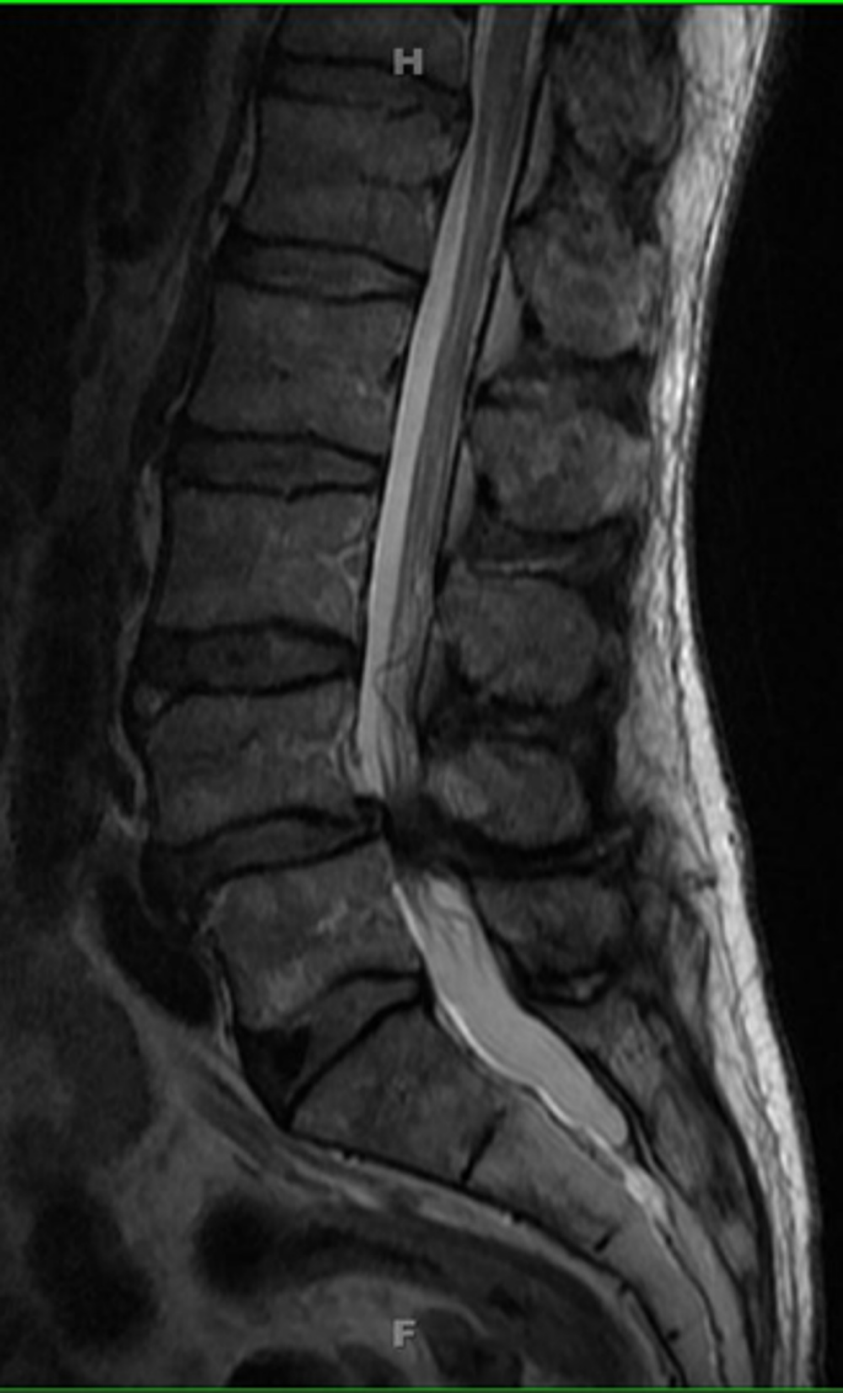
MRI, sagittal T2 MRI imaging reveals severe multilevel spinal stenosis with severely hypertrophied ligamentum flavum
